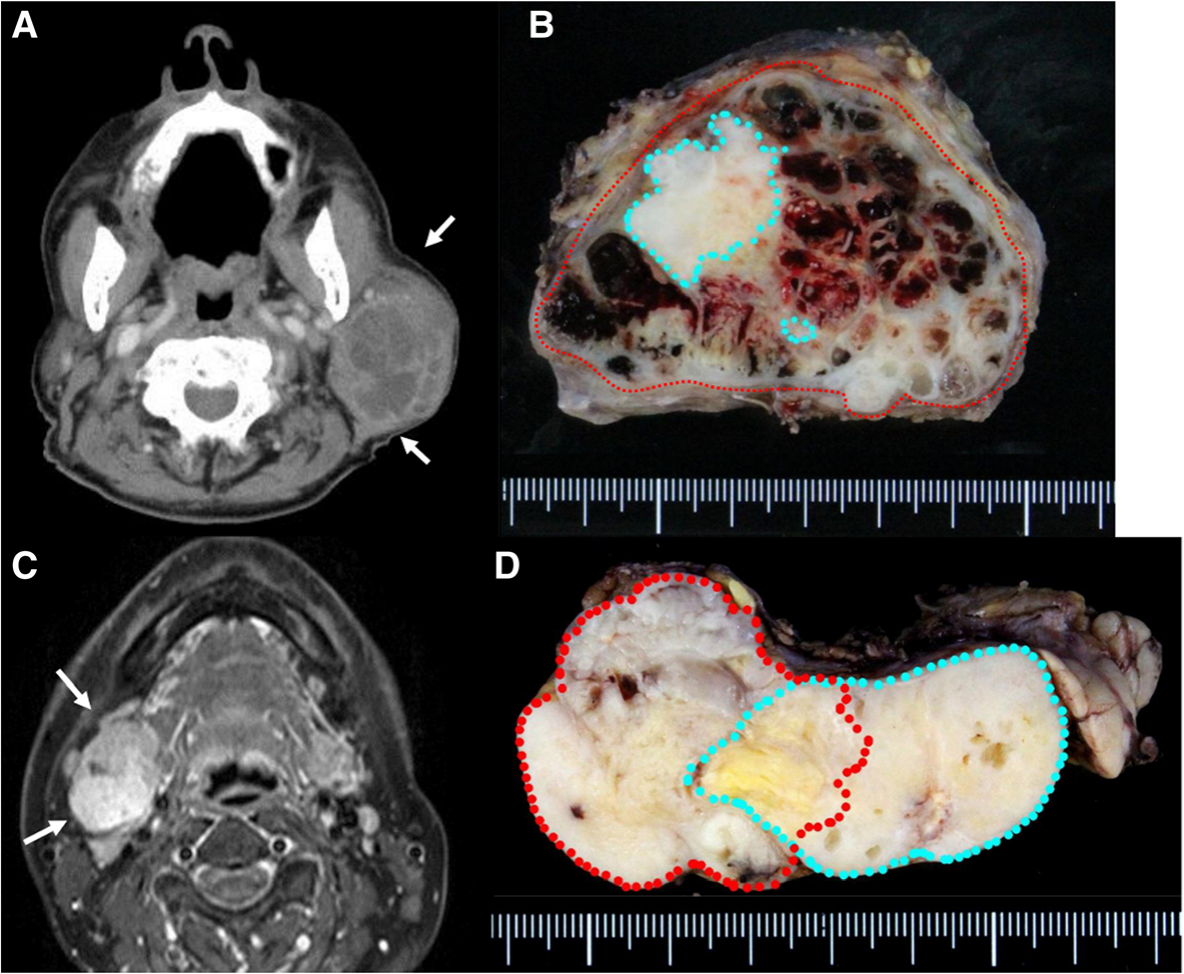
Enhanced CT revealed a large left neck mass with partial enhancement. Necrosis of the inner tumor was suspected. a Macroview of the tumor: PA was completely surrounded by MEC. *Red line*, cancer; *blue line*. PA, b MRI revealed a mass with bumpy surface in the right submandibular gland with a partially unclear border. c Macroview of the tumor: MEC existed outside of PA. *Red line*, cancer; *blue line*, PA
